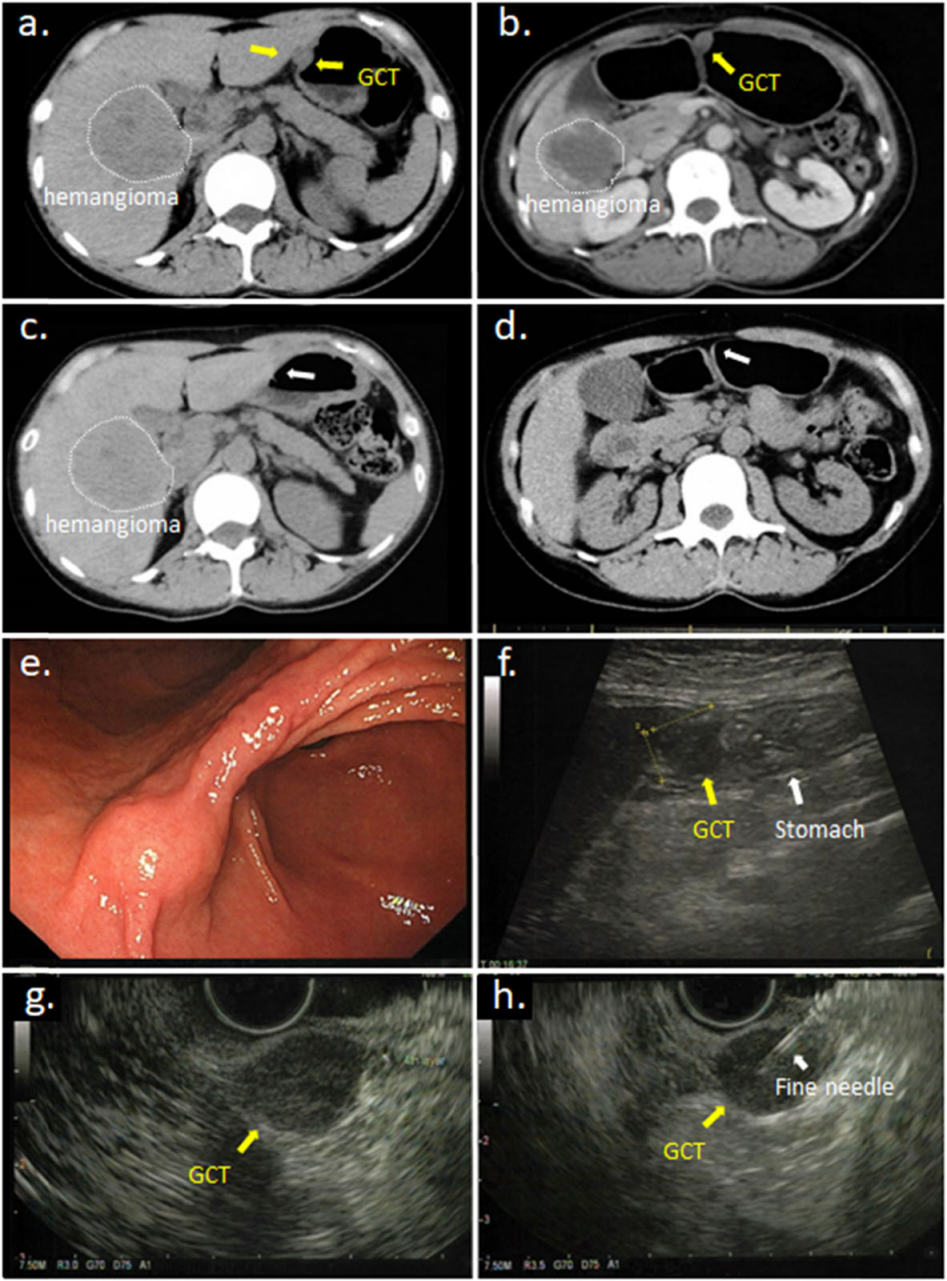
a Abdominal computed tomography (CT) revealed a solid extramural tumor situated at the lesser curvature of the lower body of the stomach (yellow arrow). The portion surrounded by white dotted line is hepatic hemangioma. b CT using foaming agent showed it more clearly. c The CT scan performed one year ago and d 4 years ago showed the tumor did not exist (white arrow). e Gastroscopy revealed submucosal tumor at the anterior side of the angle of the stomach. f Abdominal ultrasound sonography showed a low echoic mass measuring 15 mm in diameter existed in contact with the stomach. g Endoscopic ultrasound sonography (EUS) showed that the tumor of about 15 mm in diameter occurred from the fourth layer of the gastric wall and h the needle of EUS-guided fine needle aspiration biopsy (EUS-FNA) surely punctured to the tumor
